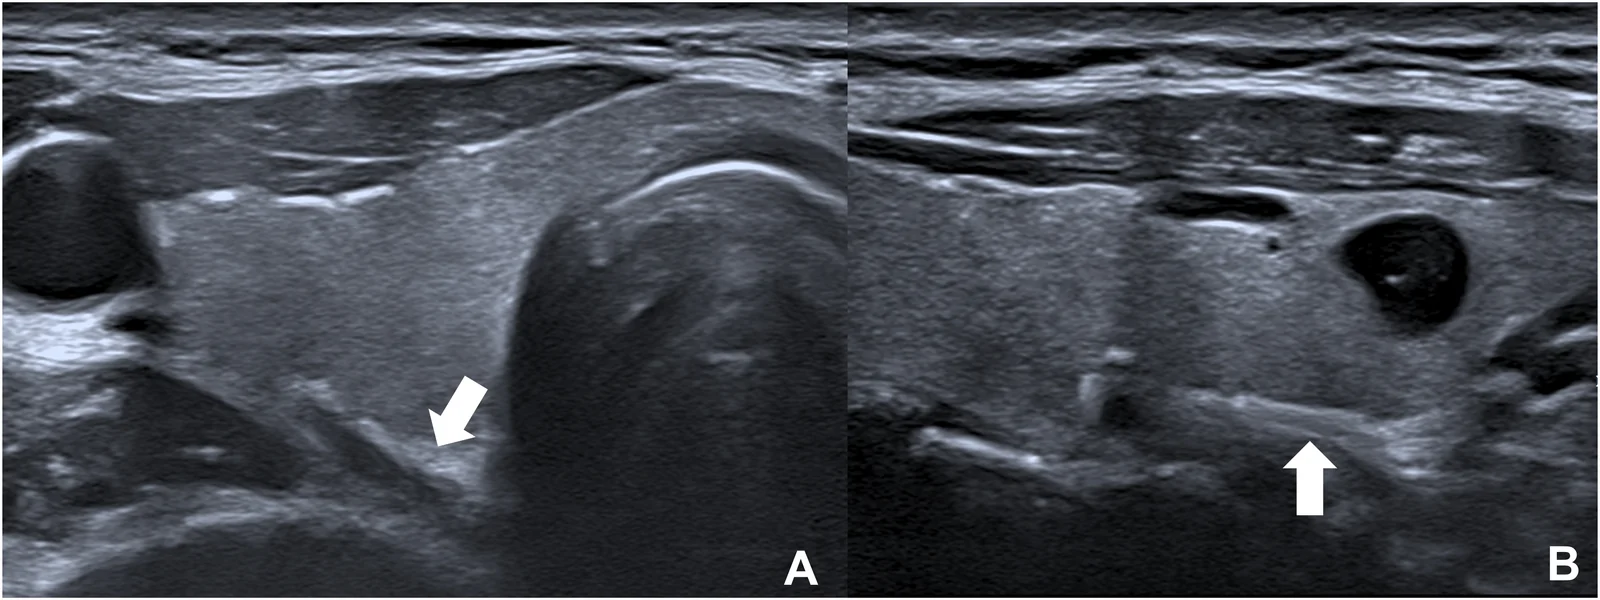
Ultrasound appearance of the right recurrent laryngeal nerve (RLN). (A) Transverse ultrasound image showing the RLN (white arrow). (B) Longitudinal ultrasound image showing the RLN (white arrow) along its course. Original images acquired at our institution.
